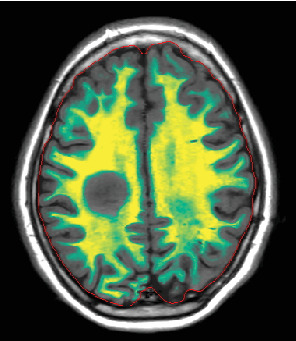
Axial image from synthetic MRI myelin maps (on the day of admission) reveals a uniform “ring of decreased myelination” surrounding the lesion.
